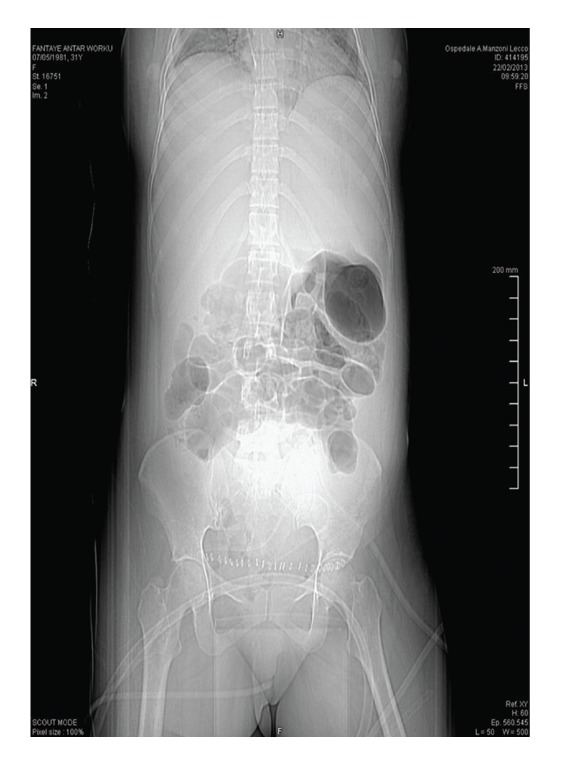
CT scan:* intraparenchymal hepatic hematoma with active bleeding from the right and left ramus of hepatic artery. A concomitant subcapsular hematoma with an extra capsular caudal extension in the paracolic gutter.
